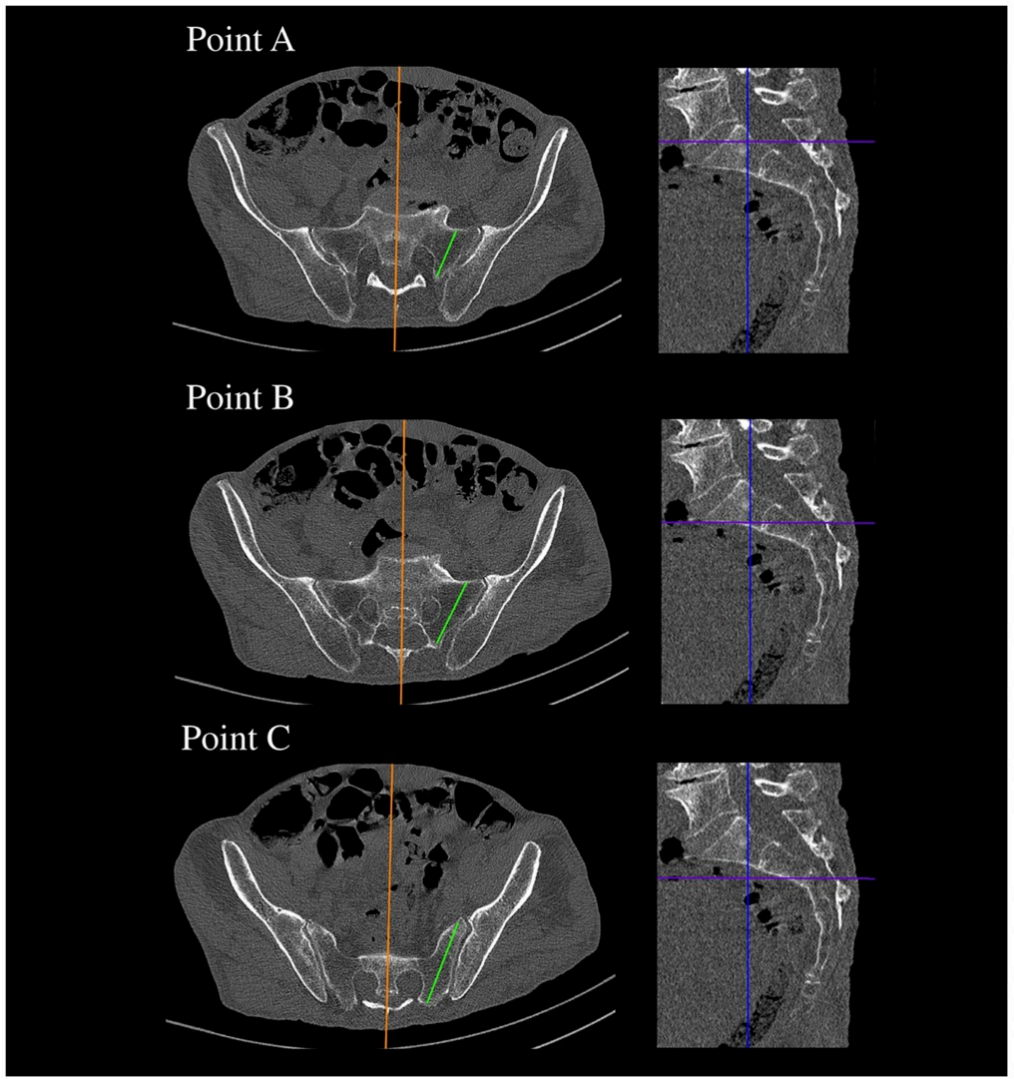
CT showing the sagittal distance between the pelvic and dorsal surfaces of sacrum that corresponds to the length of a screw, which can be applied at each level.
